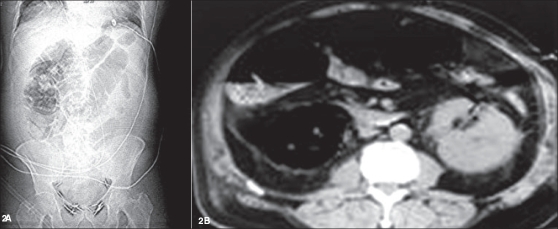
A plain abdominal X-ray examination (Figure 2A) suggests and a transaxial computed tomogram (Figure 2B) confirms, the presence of gas in the right kidney. The computed tomogram also shows a small amount of gas in the left kidney. (Figure 2B portrays an inferior view of a cross-section of the torso)
